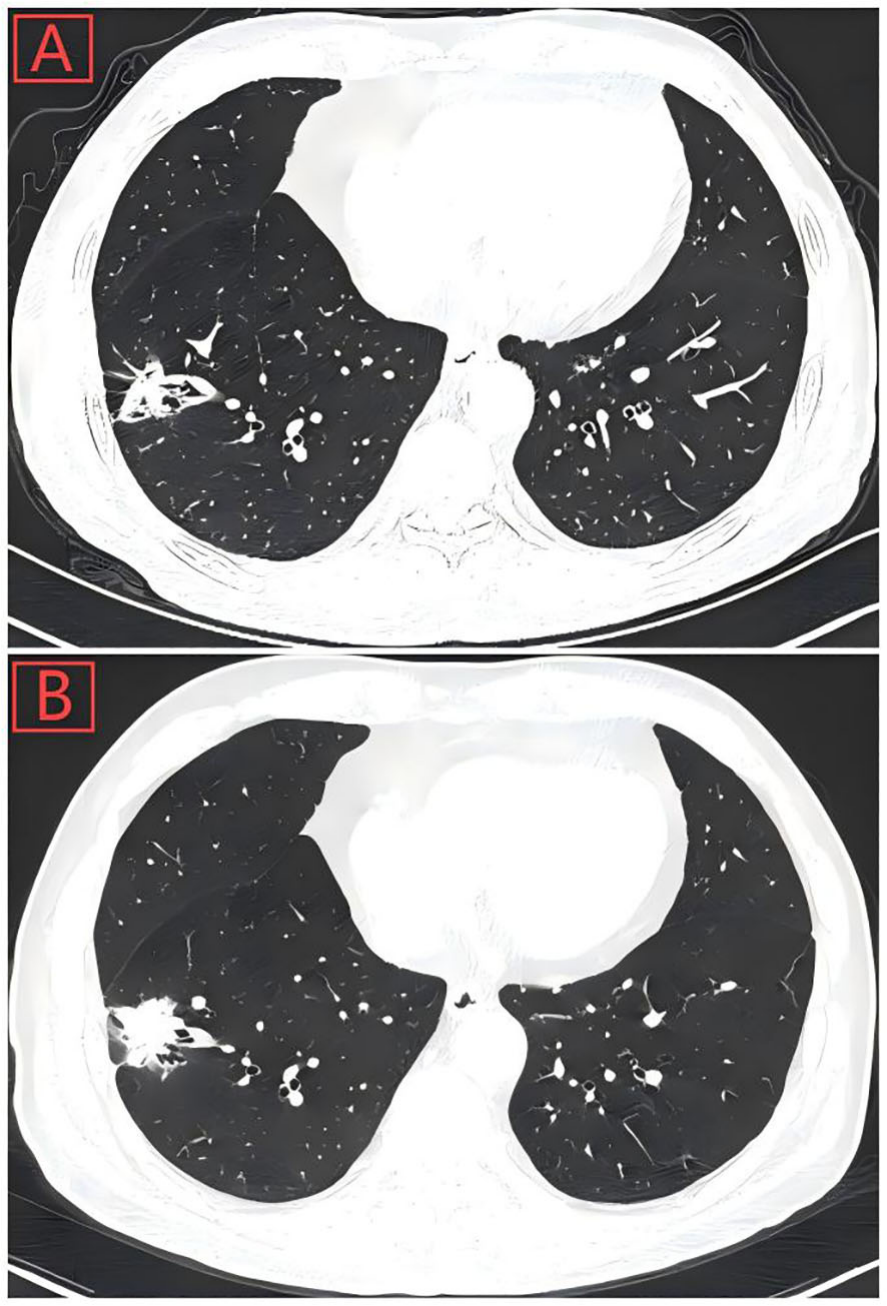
(A) Thoracic computed tomography scan showed a pulmonary nodule with solid opacity (28-mm diameter) on March 16, 2024, located in the peripheral aspect of the right lower lobe. (B) Pulmonary nodule had increased in size (35-mm diameter) by follow-up Chest CT on October 4, 2024.
